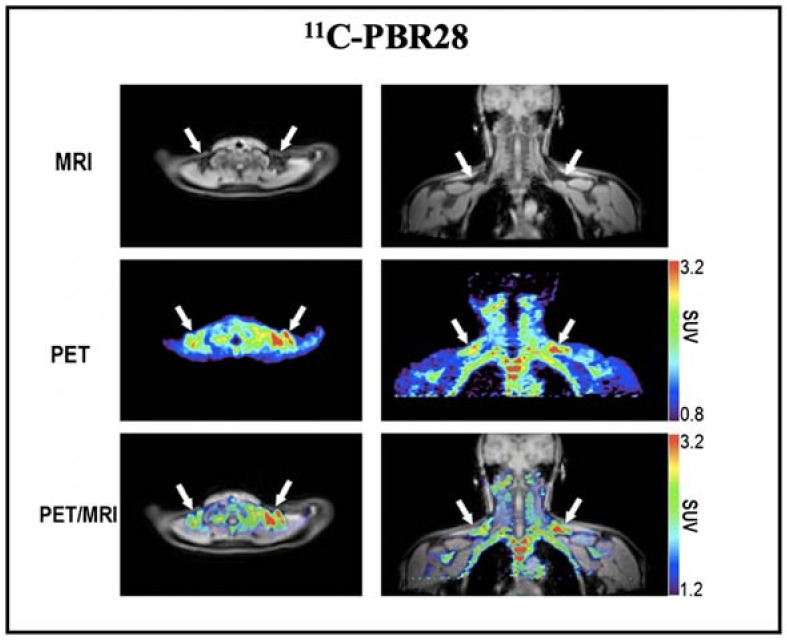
BAT imaging with TSPO PET tracer: ^11^C-PBR28. Representative images of a healthy volunteer who underwent PET/MRI using ^11^C-PBR28. The images were obtained 60–90 min after 14 m Ci of ^11^C-PBR28 injection. The supraclavicular BAT depots are indicated with arrows. Reproduced data with permission from reference [64].
